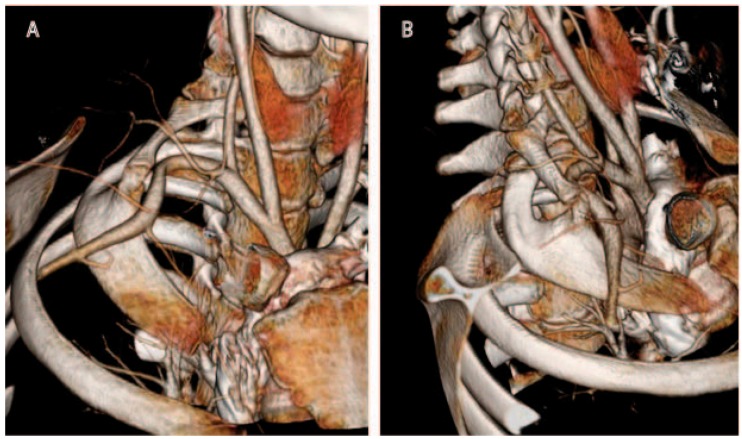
3D reconstruction of computed tomography (CT) arteriography, demonstrating a right-sided cervical rib fused to a broadened first thoracic rib, with anterior displacement of the subclavian artery. (A) antero-posterior view; (B) oblique view.
